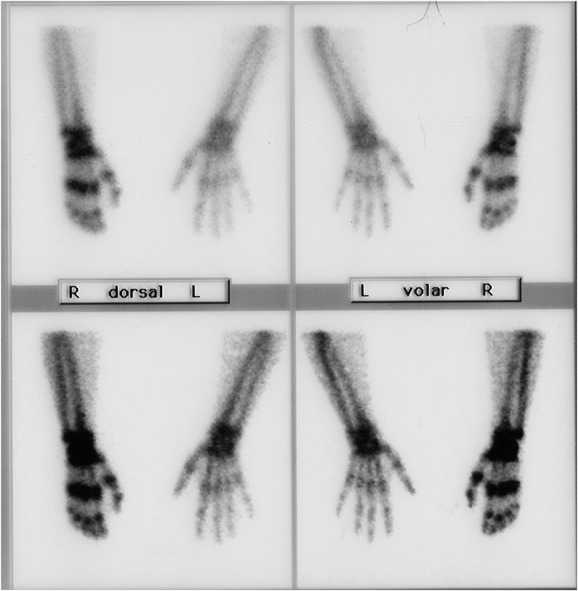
Delayed phase of three-phase bone scintigraphy. The right upper extremity shows a pathological tracer activity in the delayed phase in a strap-like manner on the wrist, the carpometacarpal bones as well as on the proximal and distal small phalangeal joints consistent with the diagnosis of complex regional pain syndrome.
